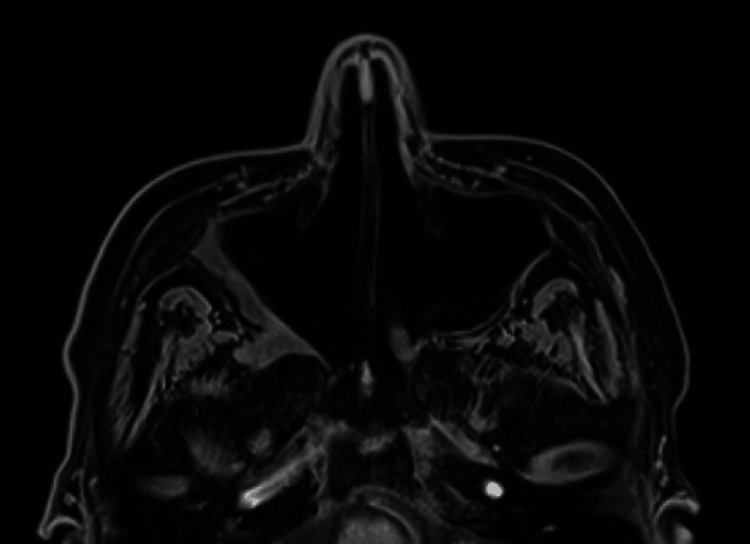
One-year post-operative MRI sinus No findings indicating invasive disease in the sinuses, indicating resolution of infection.
